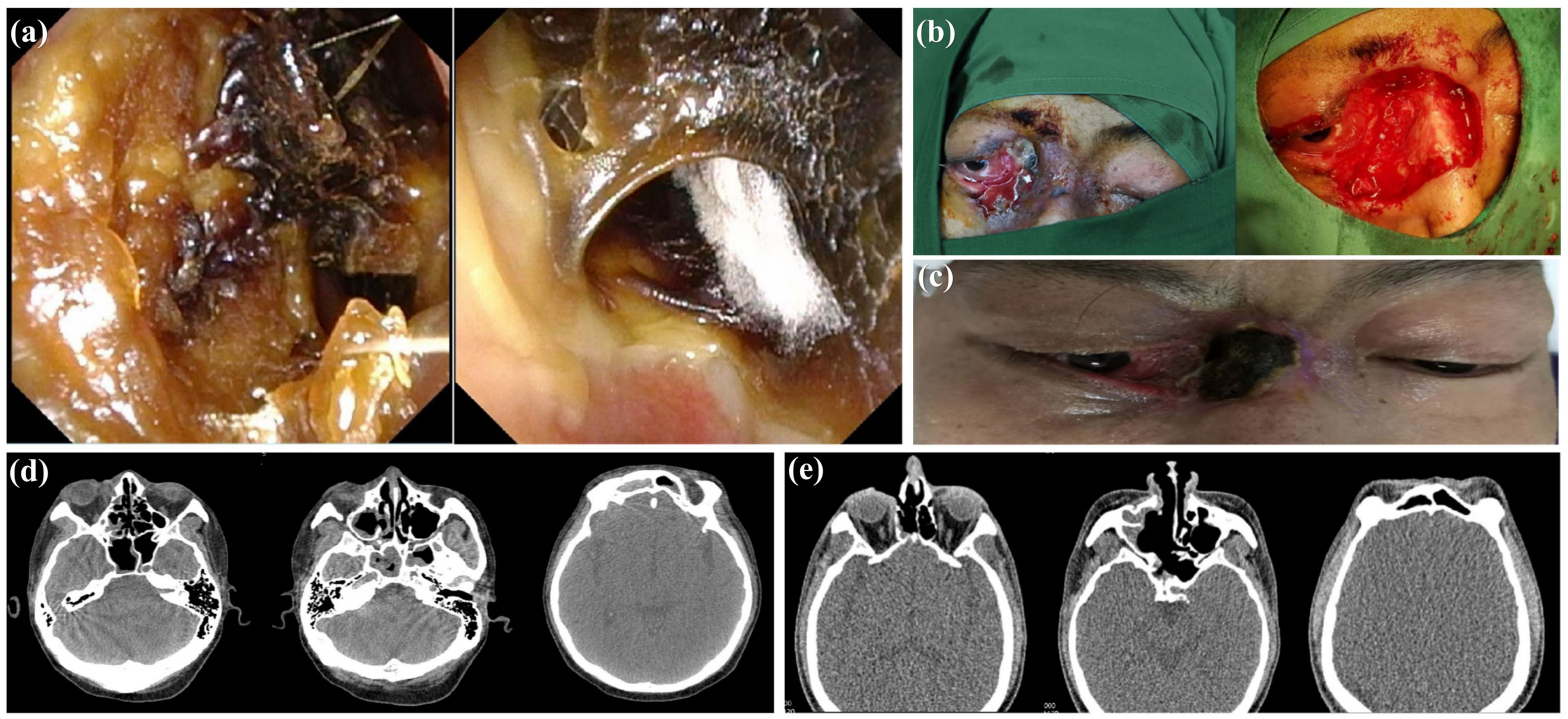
Intranasal endoscopic findings, intraoperative pathological diagnosis and preoperative and postoperative appearance of the patient. (a) Preoperative nasal endoscopy showed black crusts with white hyphae in the right nasal cavity, in line with typical features of mucor. (b) In the initial surgery, the patient’s right eyelid developed an ulcer, and the procedure involved extensive excision of the affected skin and soft tissues. (c) Good recovery, disappearance of swelling and formation of crusts on the surface skin at 3 months after surgery. (d) Preoperative sinus CT showed soft tissue necrosis in the right eye and soft tissue density shadows in the right maxillary, sphenoid, and frontal sinuses. (e) Postoperative sinus CT showed that necrotic tissue in the right eye was thoroughly cleaned and postoperative changes in the right maxillary, sphenoid, and frontal sinuses were observed.
